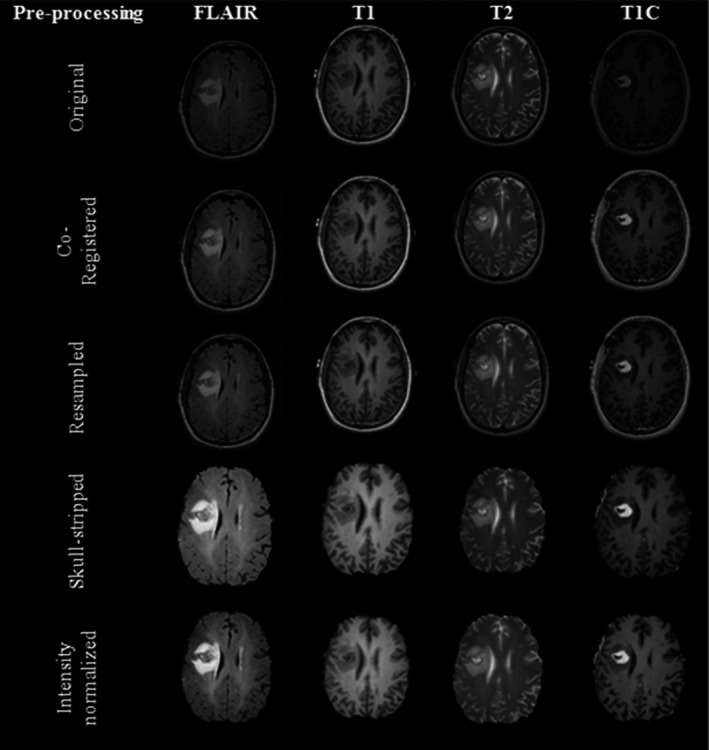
An example of our baseline preprocessing steps on a single slice of multimodal magnetic resonance imaging (MRI) (FLAIR, T1, T2, T1C) glioblastoma patient. FLAIR = fluid‐attenuated inversion recovery, T1C = Post contrast T1 weighted
